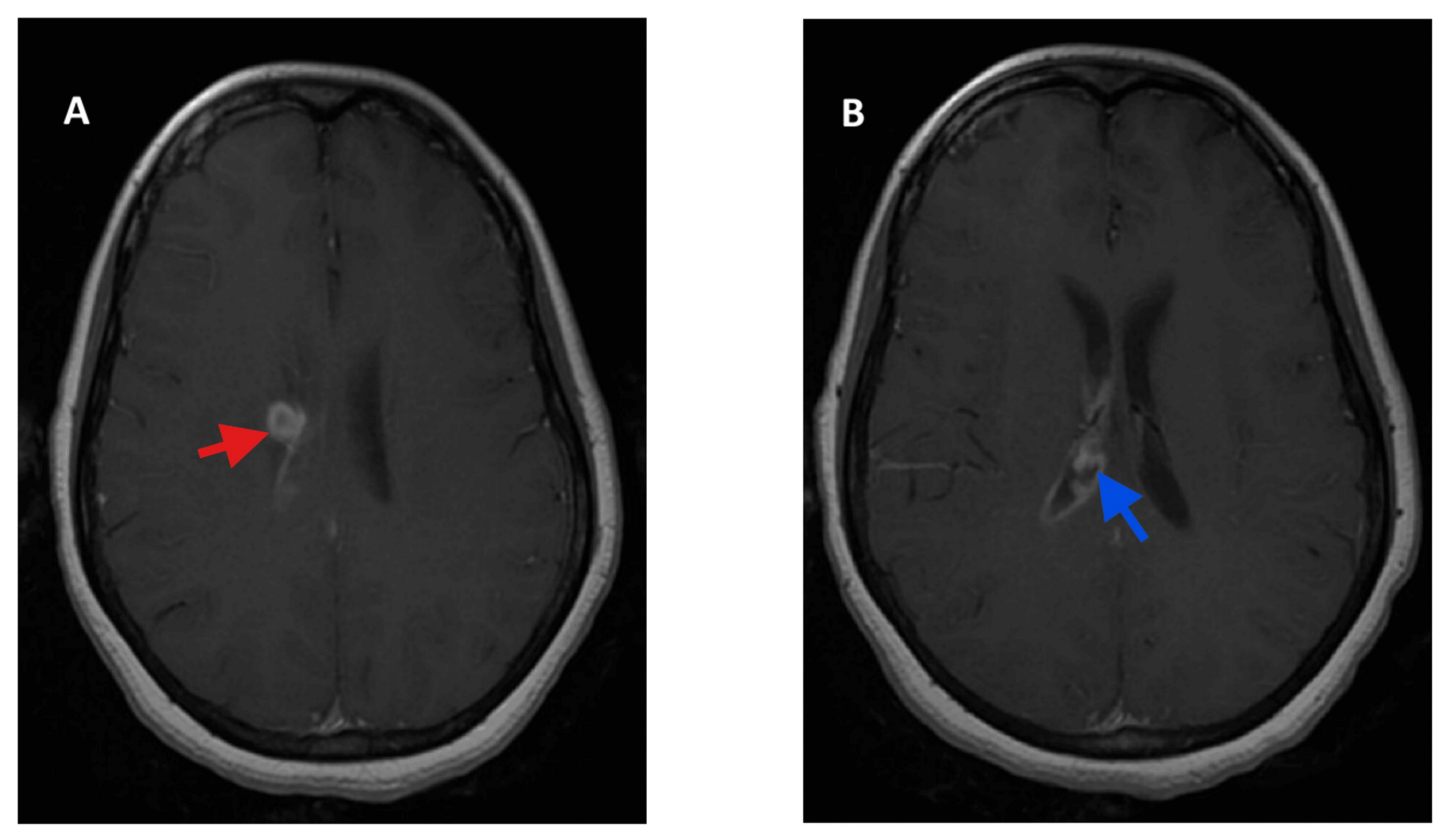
Initial MRI brain with and without contrast showing (A) 9 x 9 mm right centrum semiovale/caudate ring-enhancing lesion consistent with abscess (red arrow) and (B) debris in the right ventricle (blue arrow)
